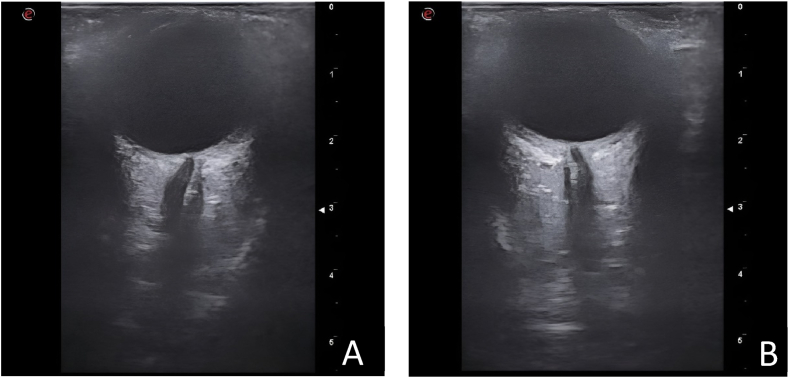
Orbital ultrasound at* 12 months*.* Ultrasonographic study of the orbit confirmed resolution of the choroidal thickening in the right eye (A) and almost complete resolution in the left eye (B) without the presence papillary protrusion.
